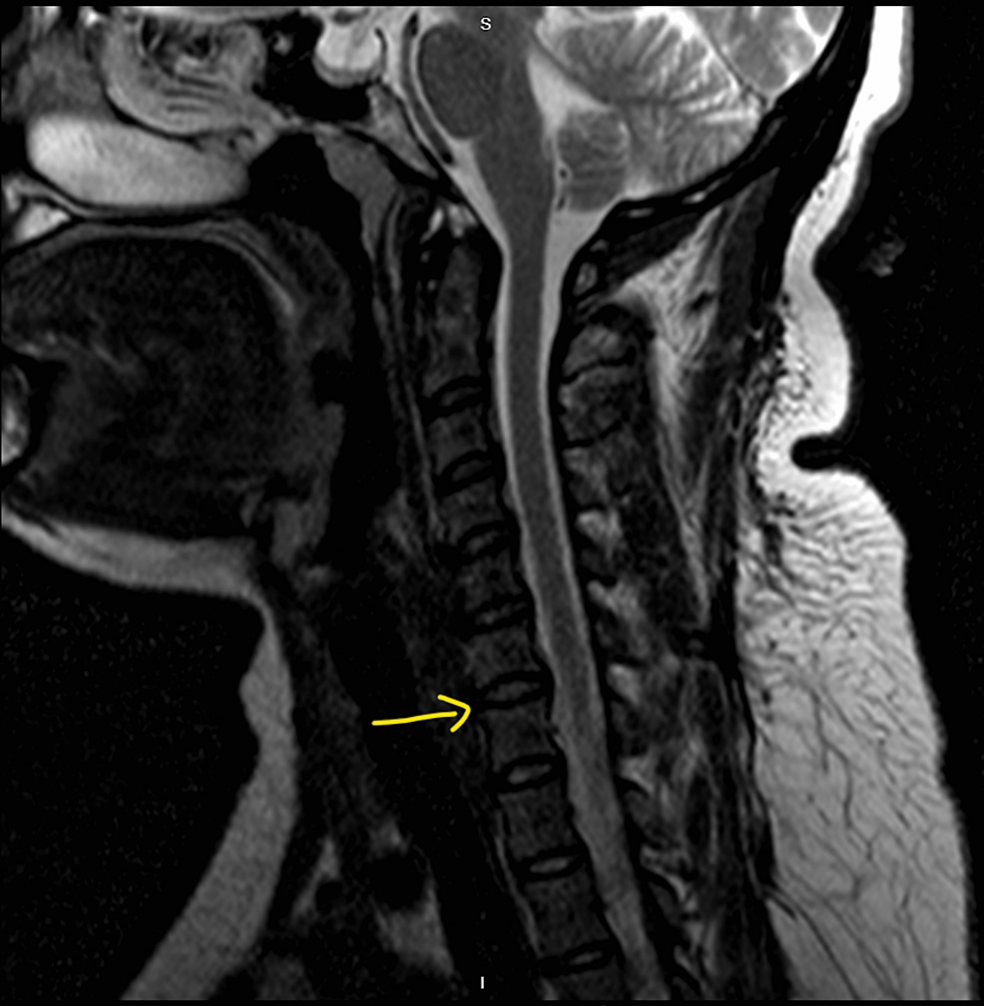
MRI of the cervical spine shows a small central disc protrusion between C4 and C5.
